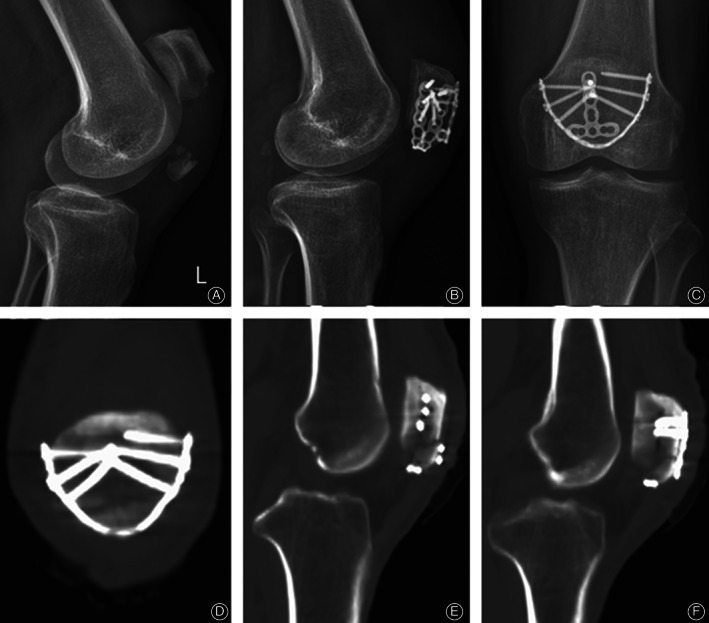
Case 2: A 48‐year‐old man suffered from an inferior patellar pole fracture of the left knee. (A) Obvious displacement of the comminuted inferior pole of the patella. (B, C) The fracture was reduced and fixed with a rim plate, with an anterior mini 2.0 locking plate to prevent anterior displacement of the distal fragments. (D–F) Postoperative CT display: the distal fragments were wrapped by the rim plate on both coronal and sagittal planes. The insertion of the patellar tendon was free from the implants.
